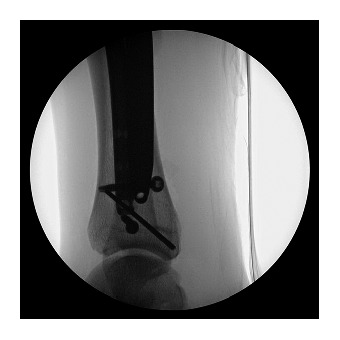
Intraoperative lateral radiograph showing placement of syndesmotic screws and the undamaged previously placed tibial nail.
